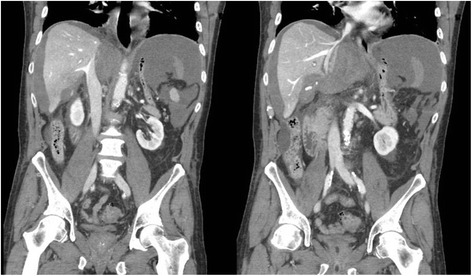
Coronal images of the computed tomography showing focal compression of the retrohepatic inferior vena cava
